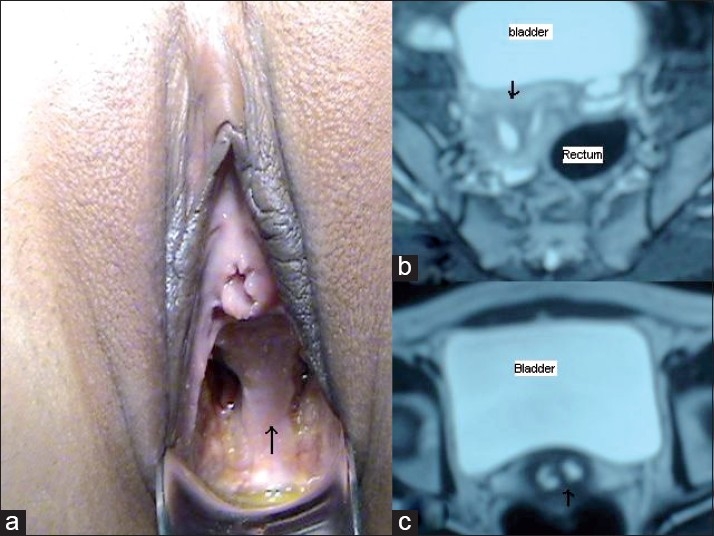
(a) Thick longitudinal vaginal septum indicated by arrow, (b) MRI showing septate uterus indicated by arrow, (c) MRI showing duplicated cervix indicated by arrow
